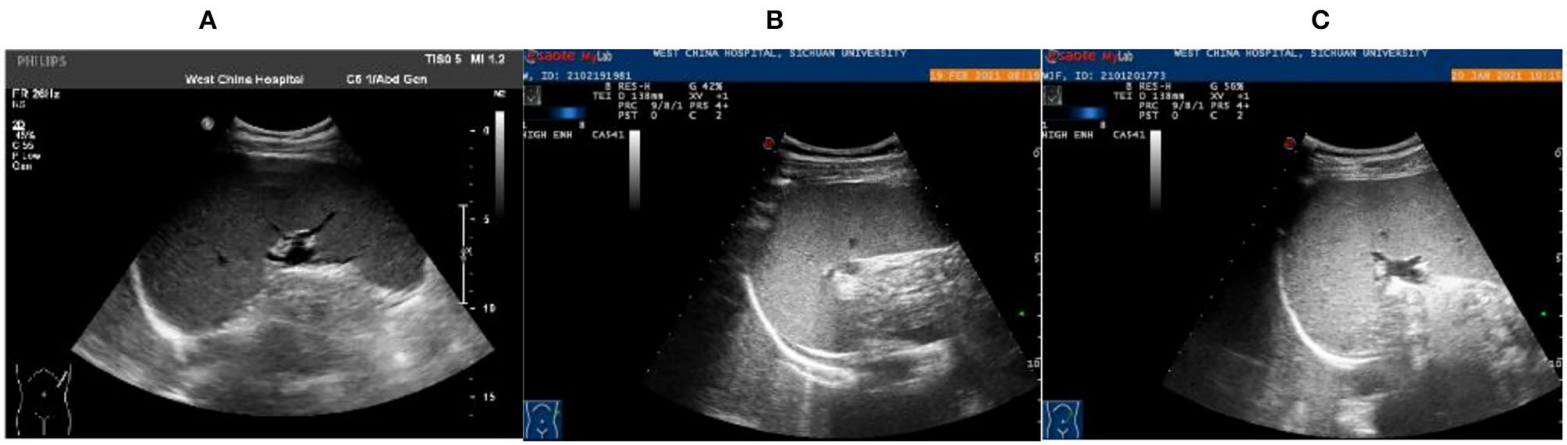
Abdominal color ultrasound of 15-year-old patient. (A) Examination results on the first day of admission showed that the intercostal spleen thickness was approximately 6.6 cm and parenchymal echo was uniform, indicating splenomegaly. (B) On the 14th day of admission, the spleen was retracted, and the thickness of the spleen was about 5.1 cm. (C) Follow-up 1 month after discharge, the spleen was retracted, and the thickness was about 4.5 cm.
